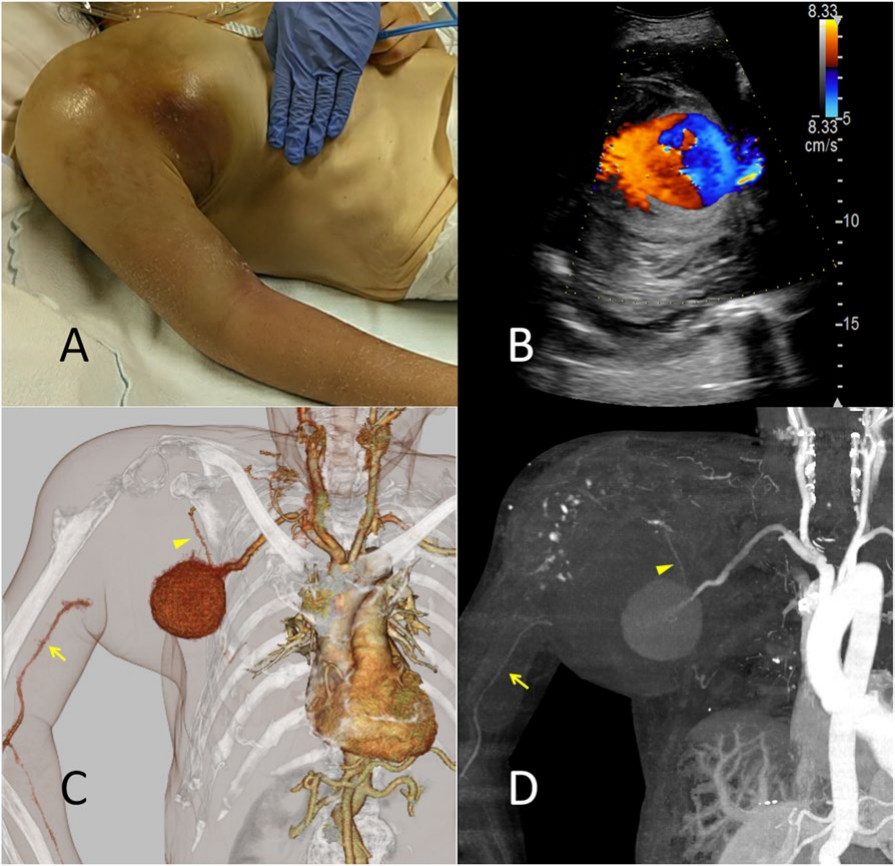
Photograph of the right swollen shoulder of the patient (A). The ‘Yin-Yang” sign detected via Doppler ultrasonography (B). The volume rendering image (C) and maximum intensity projection (D) of the pseudoaneurysm of the disrupted, right axillary artery and collateral blood-flow (arrowhead) to the right brachial artery (arrow) obtained via three-dimensional computed tomography angiography
